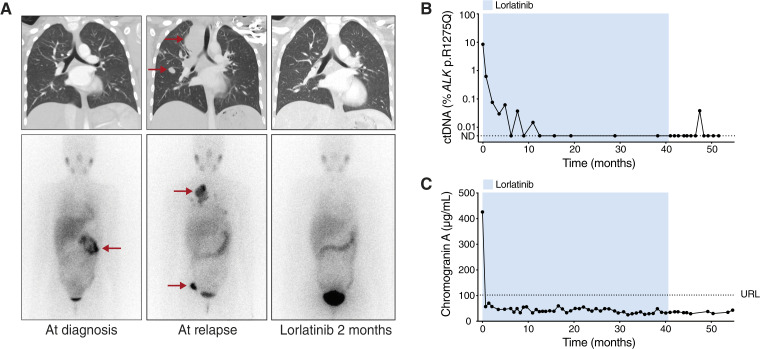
Lasting response to lorlatinib in a patient (patient 1) with relapsed neuroblastoma harboring *ALK* p.R1275Q. A, Chest computed tomography (top) and MIBG scans (bottom) at time of initial diagnosis, at relapse, and after 2 months of lorlatinib treatment. Red arrows denote tumors. B, Levels of ctDNA during and after lorlatinib treatment. ND, not detected. C, Chromogranin A. URL, upper reference limit (102 µg/L). NSE, urine VMA and urine HVA were within normal ranges at time of relapse and were therefore not further analyzed. HVA, homovanillic acid; VMA, vanillylmandelic acid.
